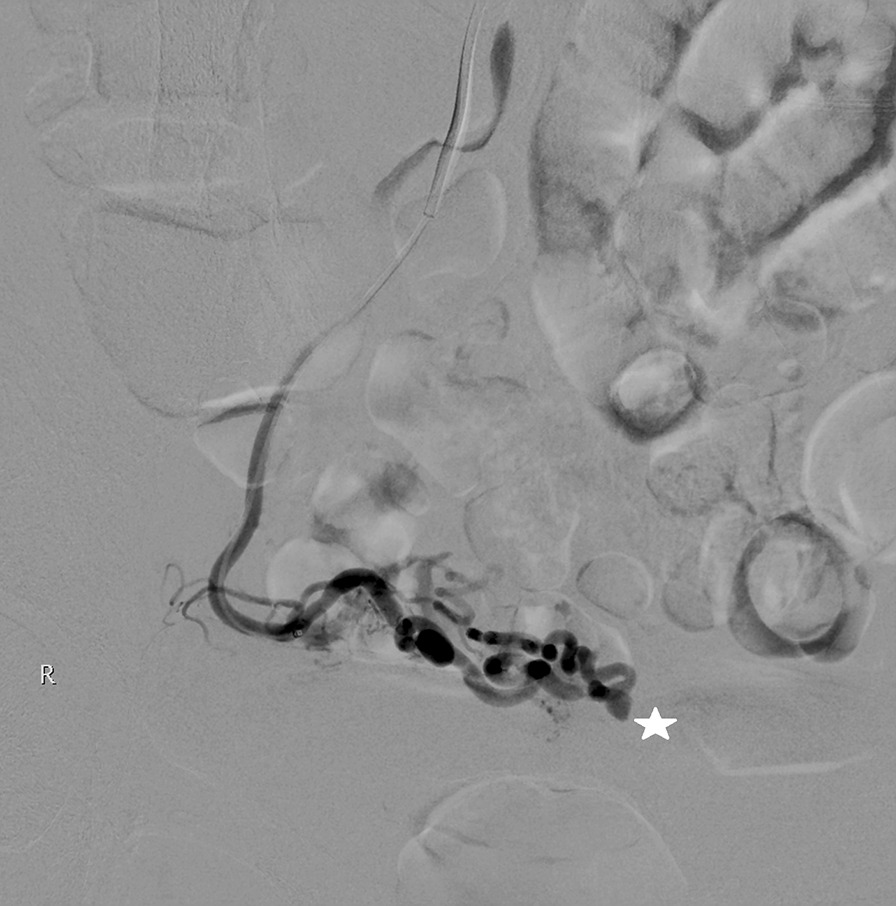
Angiogram prior to embolisation: Angiogram was performed following cannulation of the right uterine artery showing a pseudoaneurysm corresponding to the finding on the CT angiogram
